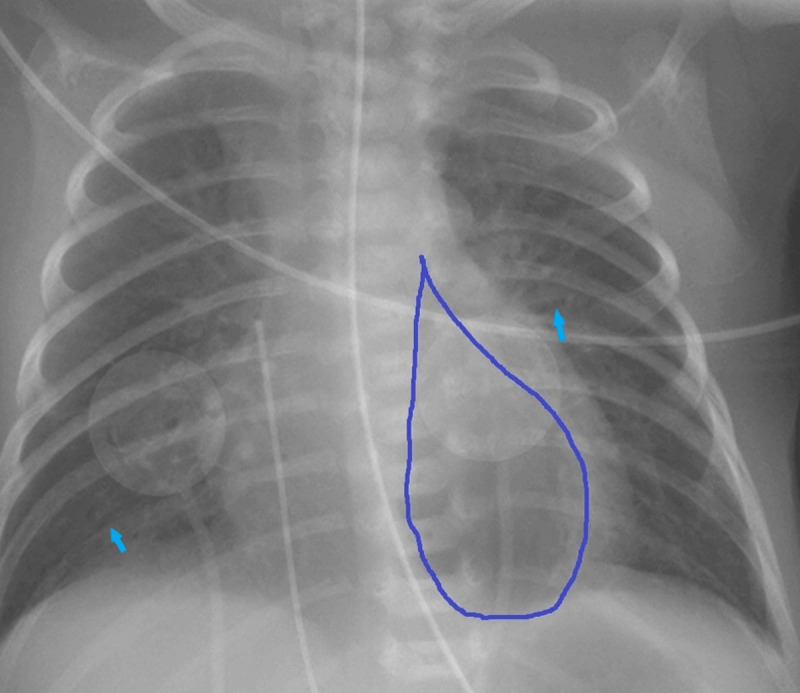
Other findings included linear radiolucent bands radiating from the hilum, consistent with pulmonary interstitial emphysema (*Figure 2*, blue arrows). There was no sign of pneumothorax. Positive pressure ventilation was diminished and a control chest radiograph performed on day 2 (*Figure 3*) demonstrated resolution of the lucency.
